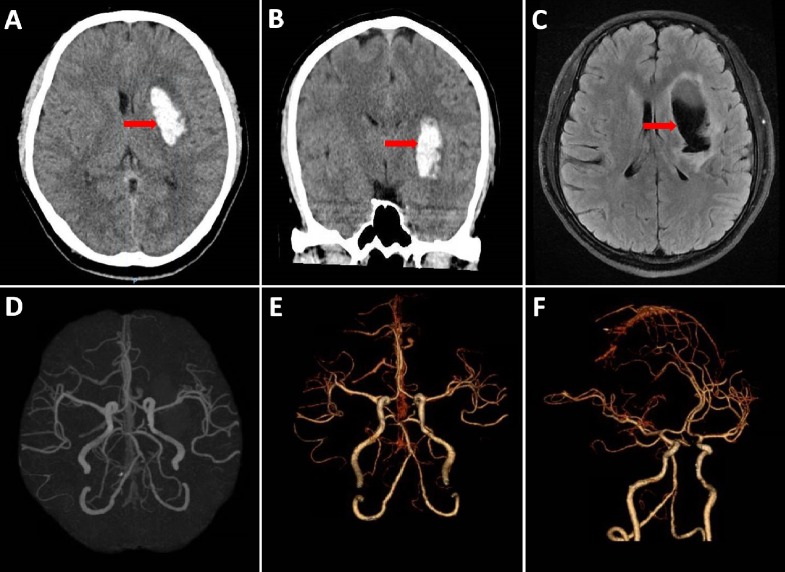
Initial Computed Tomography of Head Axial view (A), Coronal view (B) and Magnetic Resonance Imaging of Brain T2 Axial Flair (C) showing acute intraparenchymal hemorrhage (red arrow) in the left basal ganglia region. Computed Tomography Angiography of Circle of Willis (D-F) showing normal study (no aneurysm or arteriovenous malformation).
